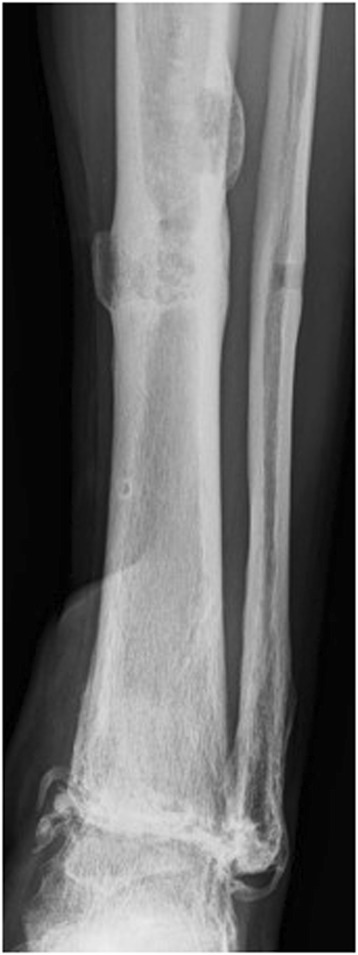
Anteroposterior radiography of the *left ankle*. There is no recurrence of osteomyelitis 2 years 8 months postoperatively
